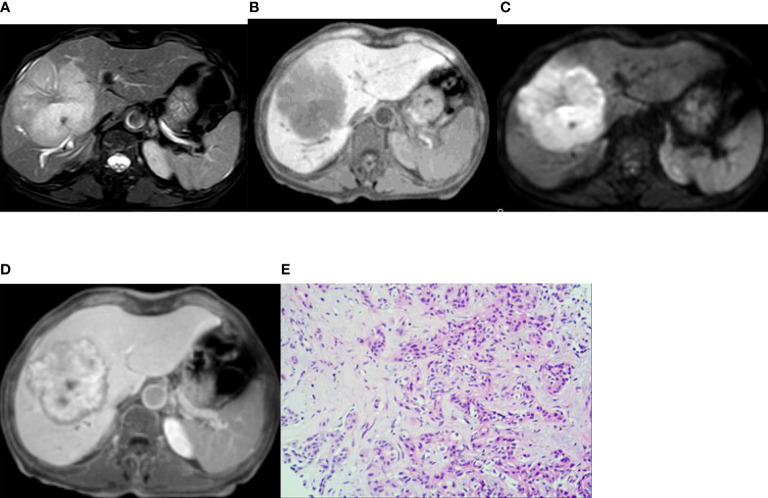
Imaging of intrahepatic mass-forming cholangiocarcinoma. A 66-year-old woman had cholangiocarcinoma with fatigue, no obvious cause of poor appetite, and laboratory examination of CA19-9 13455 U/mL. (A, B) (T2WI and T1WI) showed a lobulated mass with a long T1 and slightly longer T2 signal with clear boundaries. (C) The lesion showed a peripheral relatively high signal on DWI image. (D) On the hepatobiliary-phase enhanced imaging, the peripheral signal was low, whereas the central signal was high. The │CNR│ was 34.92 in the peripheral region but 20.94 in the central. The visibility score was 5. (E) Pathological sections showed heterogeneous epithelial cells in the fibrous tissues, some of which were glandular and in cords, with mucinous degeneration in the interstitial fibrous tissues. The pathologic diagnosis was cholangiocarcinoma (medium–poorly differentiated).
